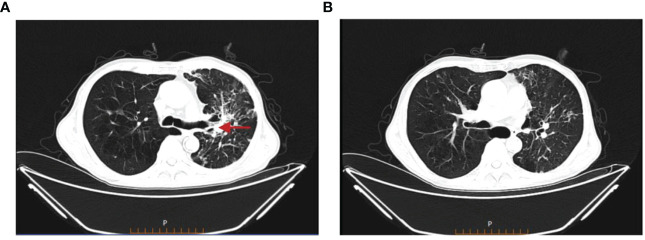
Chest CT on 19 August 2022. (A) The primary focus of cancer in the right lung was stable. (B) The nodular and small patchy high-density shadows in both lungs were less extensive than before.
